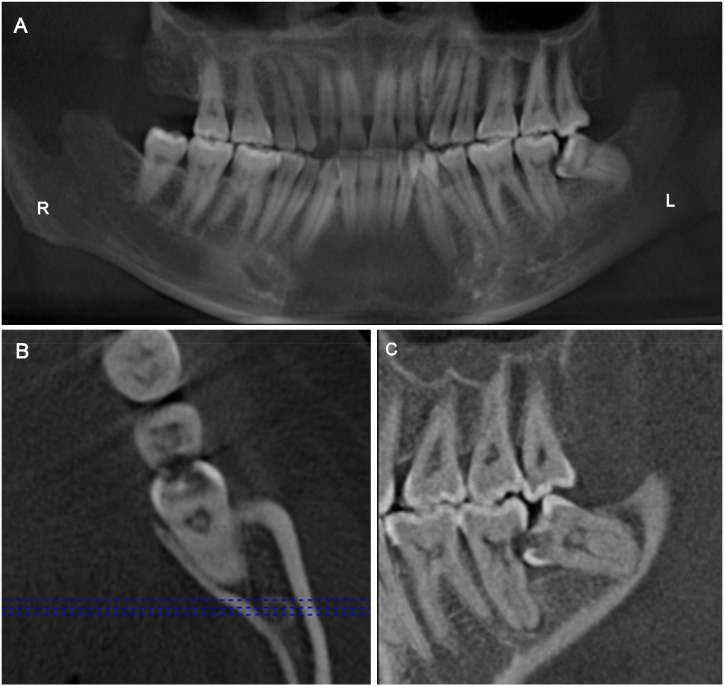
Representative CBCT images of an impacted mandibular third molar (IMTM). (A) The CBCT-generated panoramic image shows a mesioangular impaction on the left side, which is associated with carious lesions in both the mandibular second and third molars; (B) an axial section; (C) a sagittal section.
